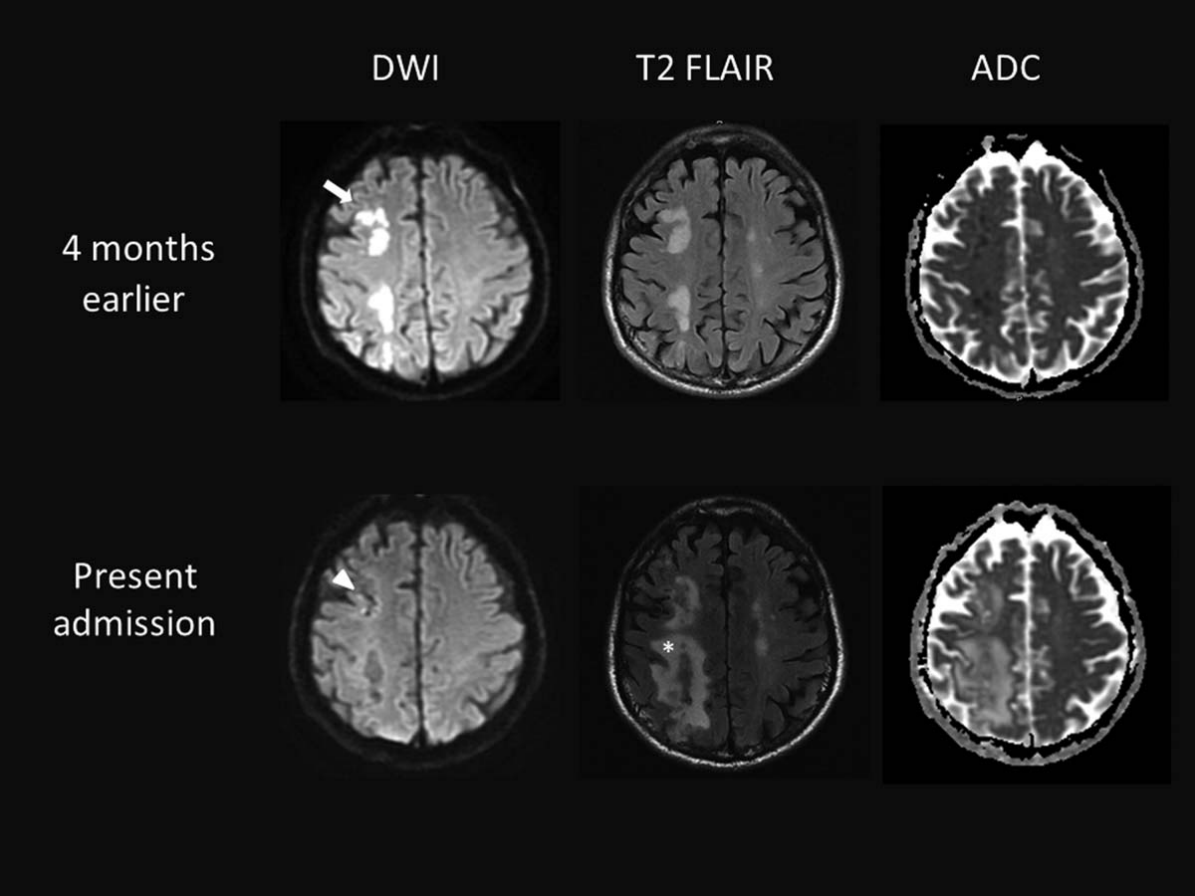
Brain magnetic resonance imaging (MRI) performed 4 months earlier revealed acute infarction in the right middle cerebral artery (MCA) territory (arrow). Brain MRI taken at the time of this admission showed multifocal increased diffusion-weighted imaging/fluid-attenuated inversion recovery signal superimposed in the right frontoparietal gyrus adjacent to the previously infarcted area (arrowhead). An increased burden of white matter hyperintensity in the right MCA territory was noted (asterisk).
